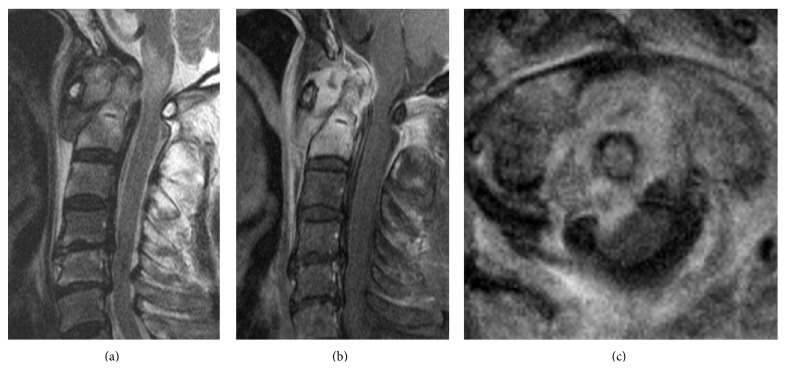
Magnetic resonance image showing abscess around the odontoid process. (a) Sagittal T2-weighted image. (b) Sagittal T1-weighted image with gadolinium. (c) Coronal T1-weighted image with gadolinium.
